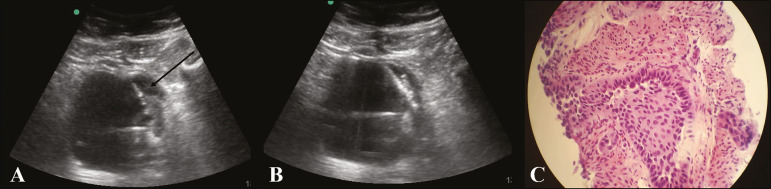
A: Ultrasound of the bladder showing a 17-G × 13-cm coaxial needle (arrow) positioned adjacent to the vegetating lesion on the left-side wall of the bladder. B: Percutaneous biopsy using an 18-G × 16-cm tru-cut needle. C: Anatomopathological examination revealed invasive urothelial carcinoma.
